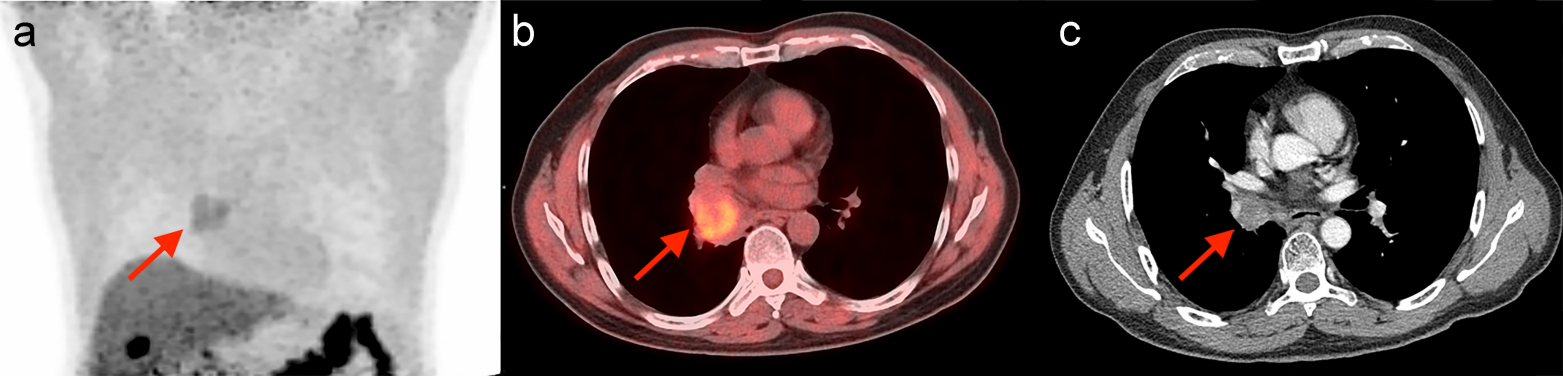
A representative case of PR prediction using the quantitative models. A 56-year-old man was pathologically diagnosed with SCC, and staged as IIIA (T4N1M0). The patient received four cycles of gemcitabine plus cisplatin chemotherapy before CIRT. The Pre-score 2 was 1.37, above the cut-off value of 0.93, and should be predicted as hypoxia. The PET/CT maximum intensity projection (MIP) (a) and axial fusion (b) images detected the single tumor (red arrow) with obvious ^18^F-FMISO uptake in the lower lobe of the right lung, with SUVmax of 3.06, TMR of 2.08, and HTV of 10.71 ml, indicating that the tumor was hypoxic. The patient received CIRT with 77 Gy/22Fx. The quantitative Pre-score 1 calculated was -1.97, below the cut-off value of -0.84, indicating a prediction of PR. Thoracic contrast-enhanced CT (c) after CIRT confirmed the tumor response as PR.
